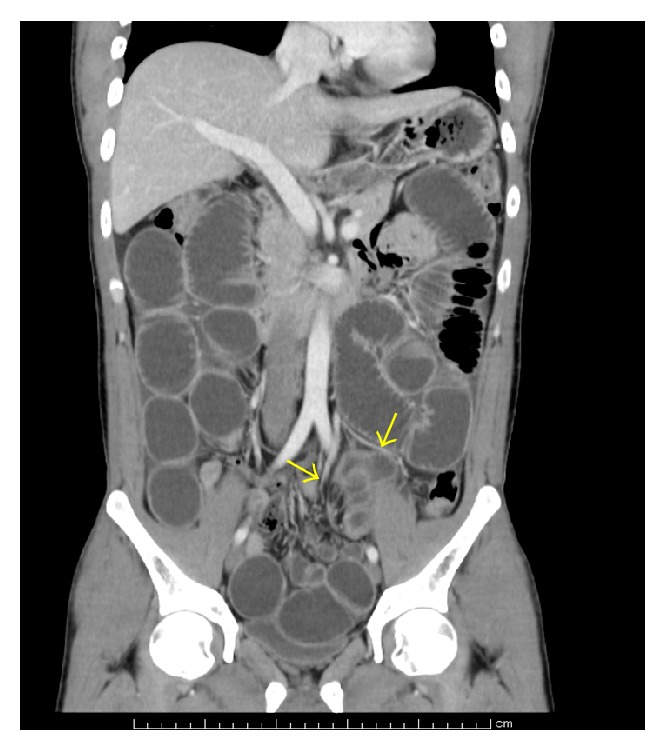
Computed tomography: abdominal computed tomography revealed an extensively dilated small bowel and a closed loop of small bowel in the mesosigmoid. The arrows indicate vessels of the mesosigmoid.
